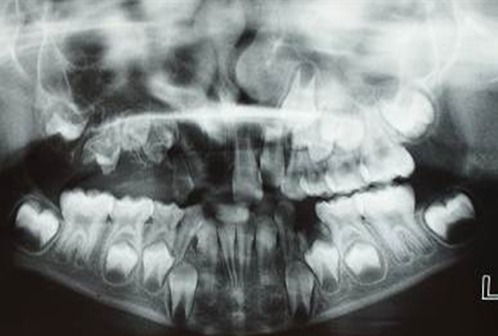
Panoramic radiograph taken during the periodic examinations
